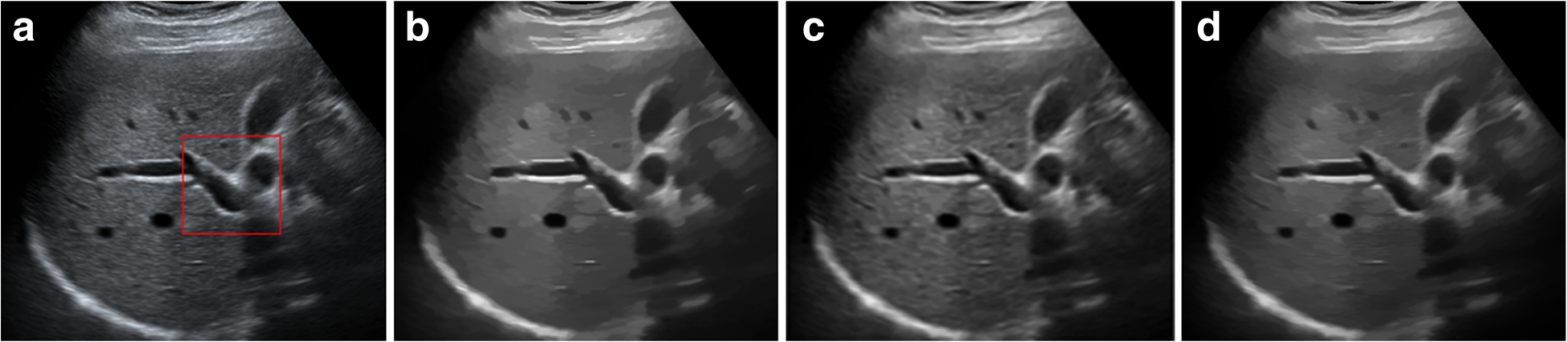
Results obtained with compared filters applied to the liver ultrasound image. a The original image. b-d Outputs of the compared filters, b BNLTV, c SAR-BM3D, d The proposed method. BNLTV = Bayesian NLTV; SAR-BM3D = blocking matching 3D filter of SAR image
